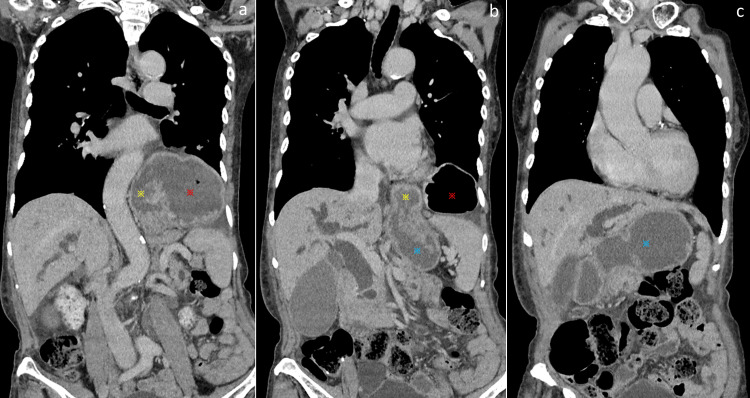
Computed tomography images a: the fundus (red asterisk) and proximal body of the stomach (yellow asterisk) are shown to have migrated into the thoracic cavity due to the giant hiatal hernia; b, c: the distal side of the gastric body (blue asterisk) is shown in the abdominal cavity.
